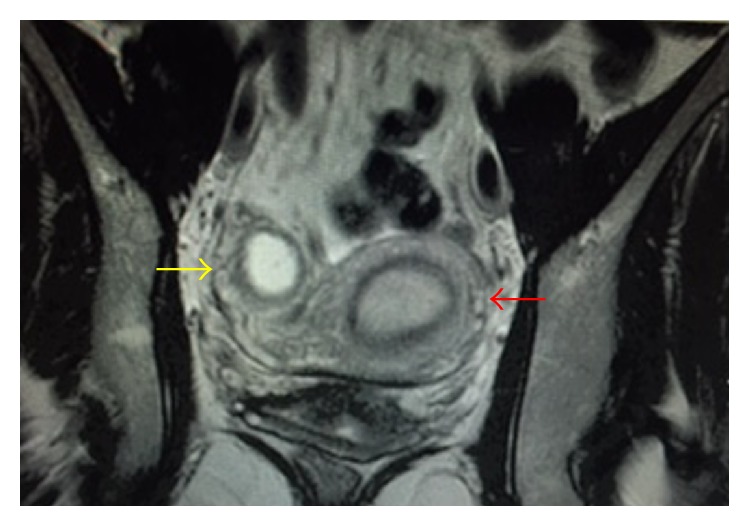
Magnetic Resonance Imaging. Red arrow: left unicornuate uterus. Yellow arrow: noncommunicating right horn with ectopic pregnancy.
